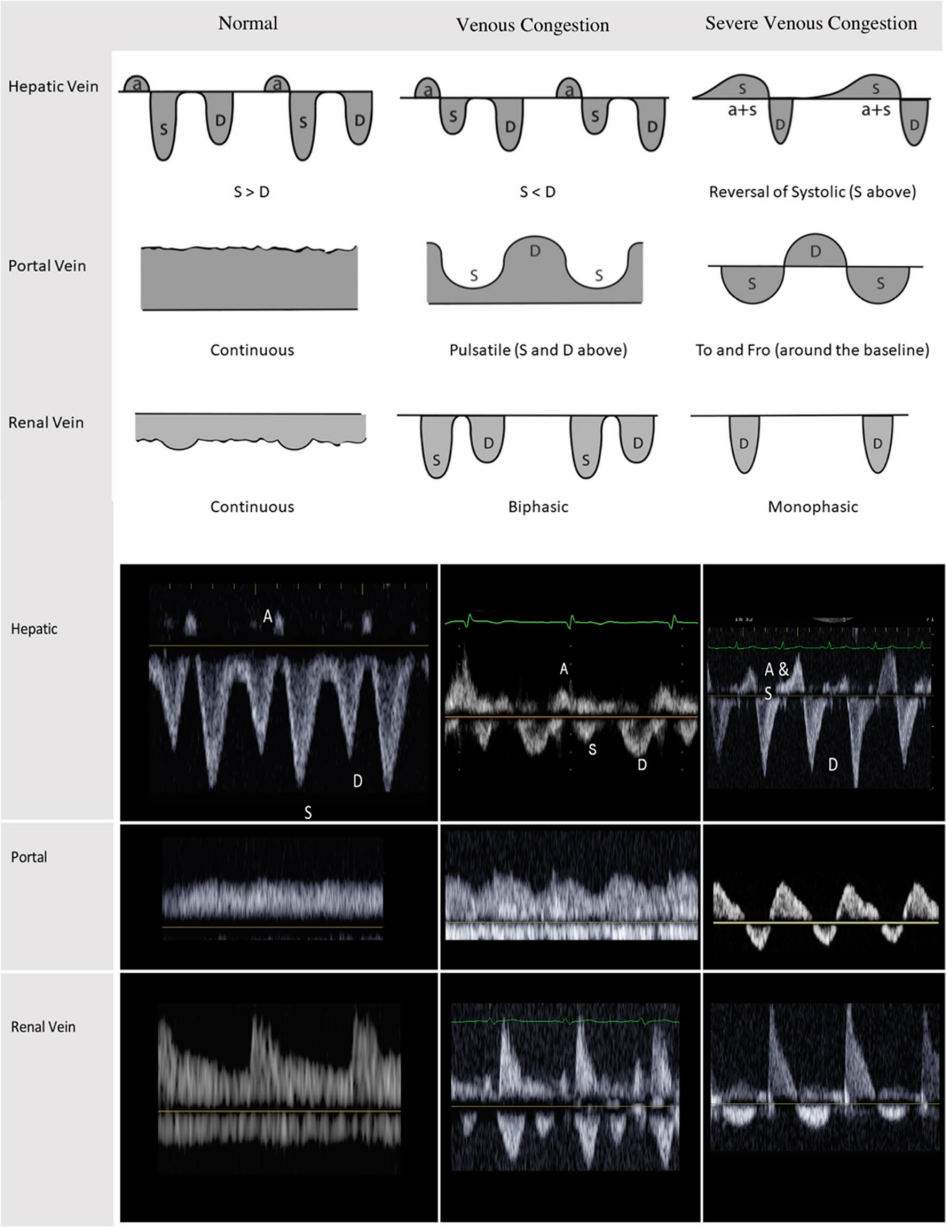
Normal and abnormal venous Doppler waveforms
